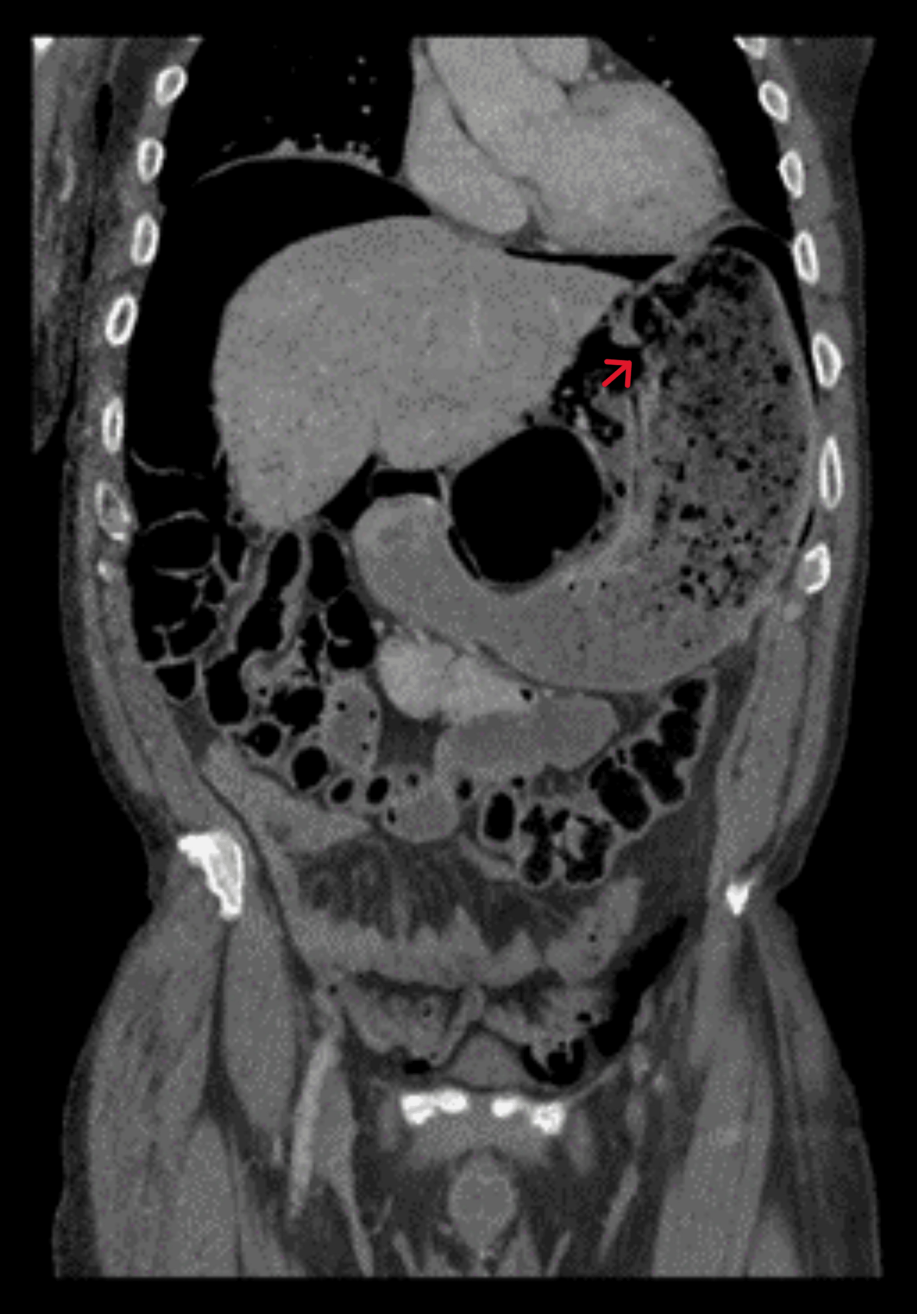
Coronal view on CT showing extensive pneumoperitoneum and discontinuity of the stomach wall (red arrow), indicative of gastric rupture
